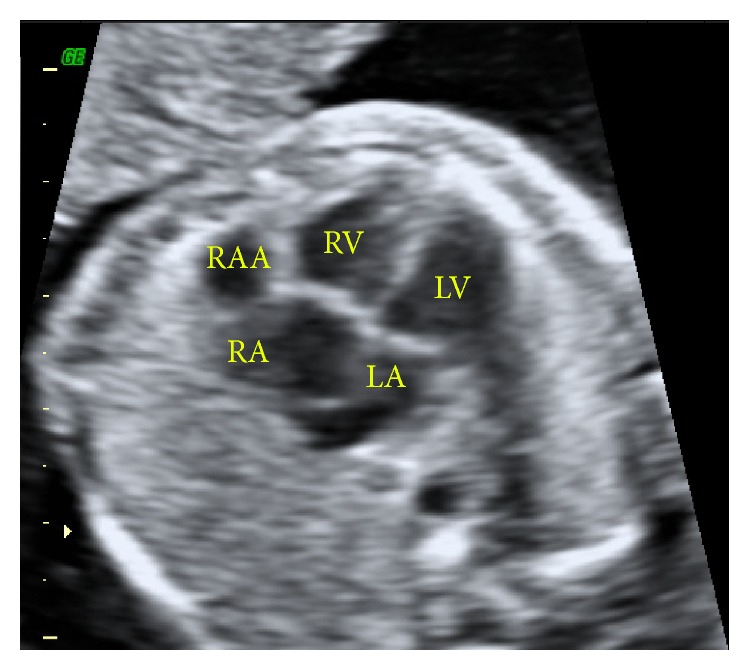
Aneurysmal dilatation of the right atrial appendage (RAA) which was detected in the 21st week of gestation with fetal echocardiography (RAA: right atrial appendage; RA: atrium; RV: right ventricle; LA: left atrium; LV: left ventricle).
